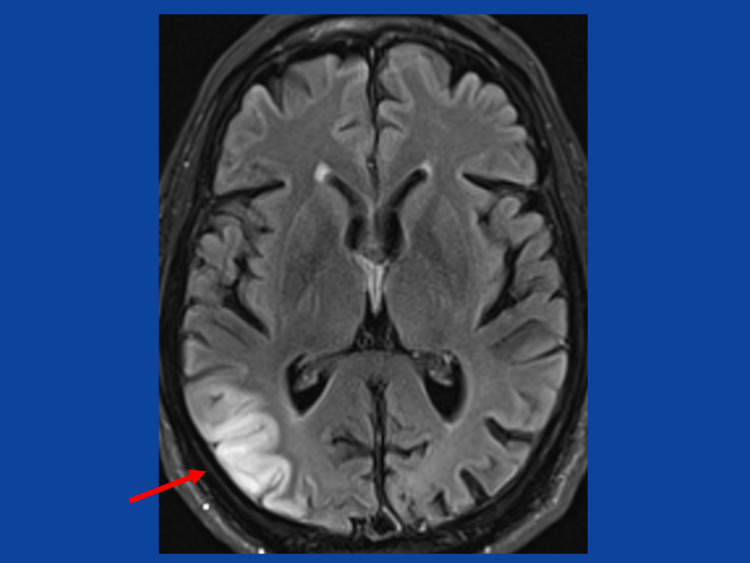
T2-weighted image showing a stroke-like lesion in a right parieto-occipital distribution
